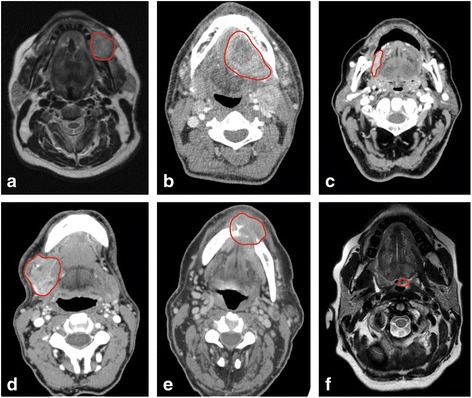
Primary tumors of six patients encircled in *red*. a Axial T1 MRI image of a tumor in the left mandible of patient 1. b Axial ceCT image of a tumor in the floor of mouth of patient 2. c Axial ceCT image of a tumor in the right lateral tongue of patient 3. d Axial ceCT image of a tumor in the right mandible/floor of mouth/tongue of patient 4. e Axial ceCT image of a tumor in the floor of mouth in patient 5. f Axial T1 MRI image of tumor in left mid tongue base of patient 6. ceCT = contrast enhanced computed tomography
